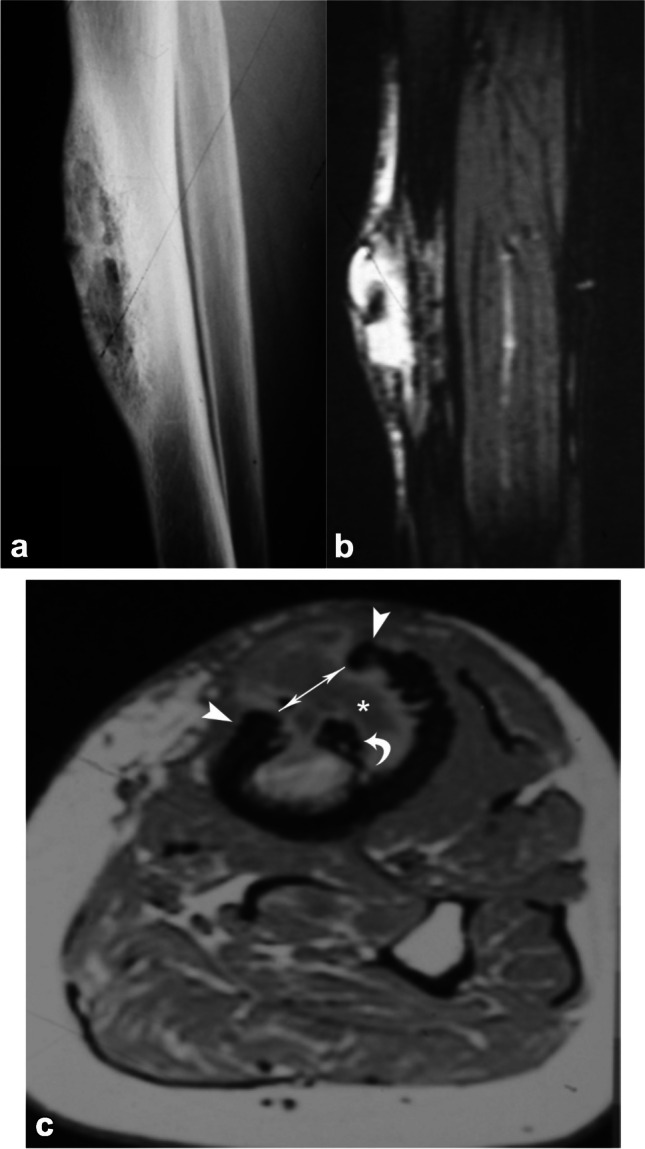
Tuberculous osteomyelitis of the tibia. Lateral radiograph (a) demonstrates expansile lytic lesion of the anterior cortex of the mid tibial diaphysis with corresponding fluid signal intensity and adjacent bone marrow edema on sagittal STIR MRI (b). Axial T1 MRI (c) shows intraosseous abscess (*), sequestrum (*curved arrow*), cloaca (*double headed arrow*), and involucrum (*arrowheads*) with the abscess extending into the soft tissues through the cloaca
